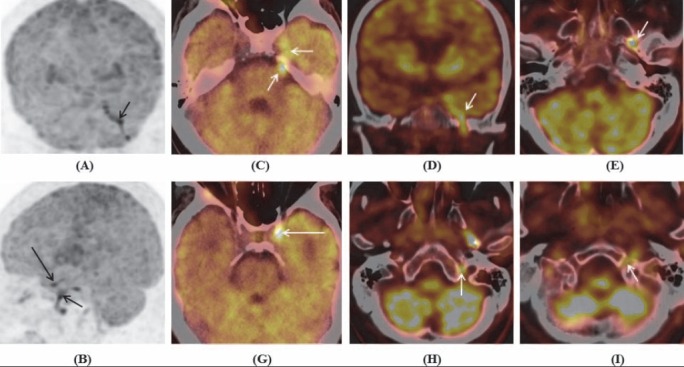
Maximum intensity projection of brain coronal (A) and sagital (B), and PET/CT images of brain (C-I) showed increased FDG uptake in the left cranial nerves: (A-E) Cranial nerves V and V3; (B, G) complex of cranial nerves III, IV, VI, V1, V2 at cavernous sinus (long arrows); (H) complex of cranial nerves IX, X, XI at jugular foramen; and (I) cranial nerve XII at hypoglossal canal
